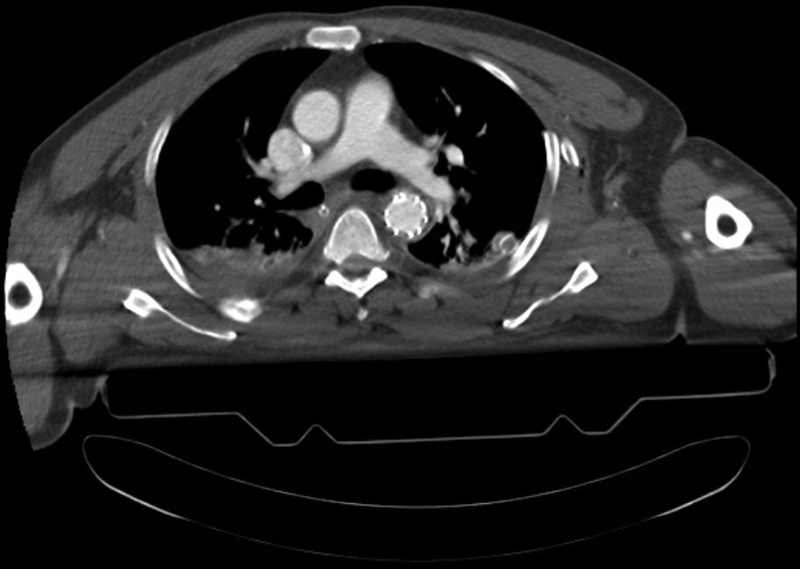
Follow-up computed tomography angiogram after endovascular repair for blunt thoracic aortic injury.
